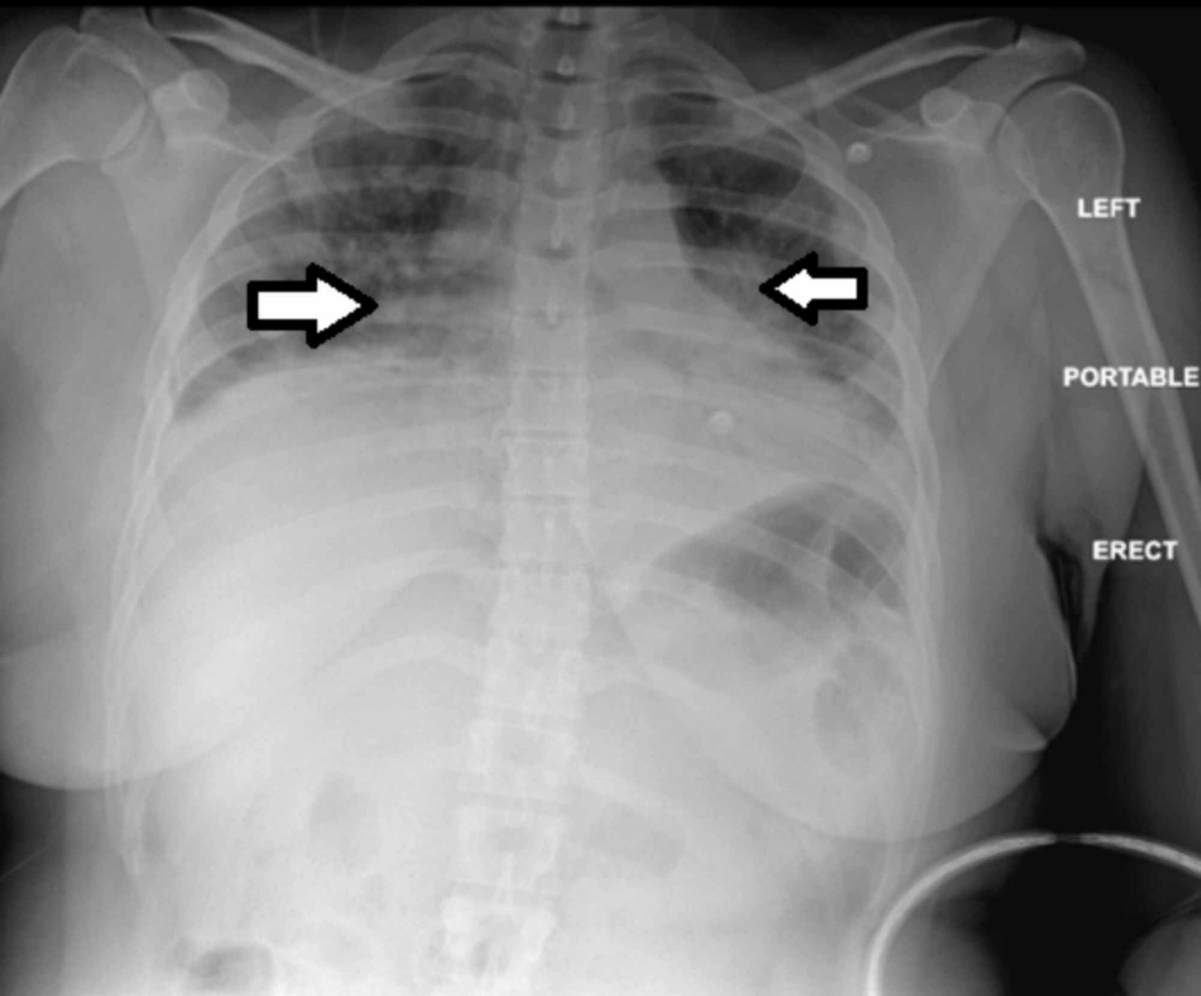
Chest X-ray of the patient at presentation to the emergency department showing low lung volumes, vascular crowding (see arrows), and bibasilar consolidations.
